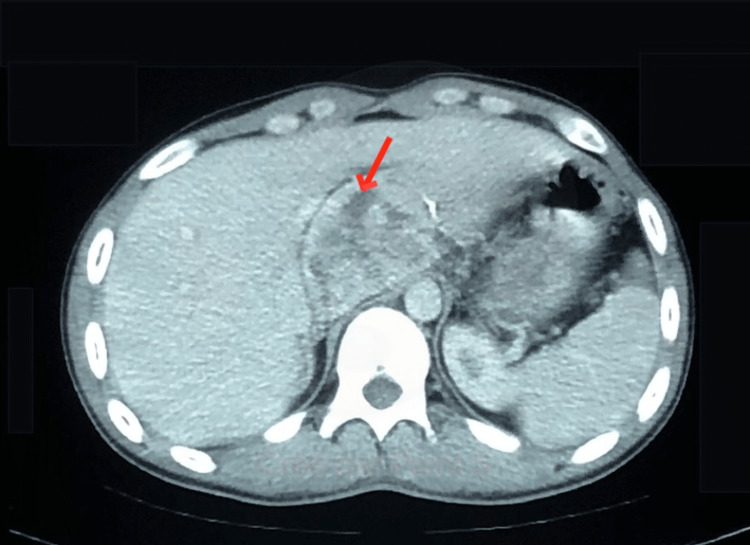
Cross section of an MRI revealing a 9.7-cm-wide retro-hepatic mass abutting the right adrenal gland MRI, magnetic resonance imaging.
